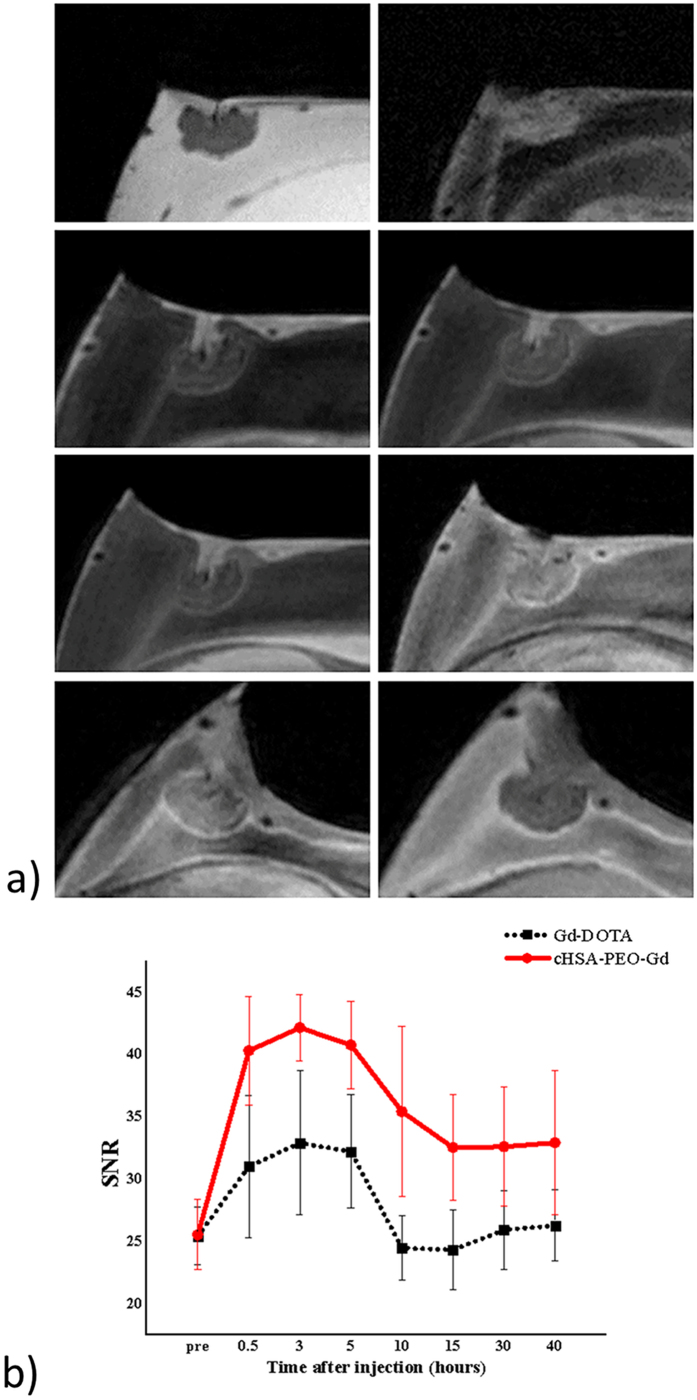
Representive MR images of cHSA-PEO(2000)_16_-Gd biodistribution in solid tumor tissuers (Left) and comparison of the SNR (Signal to Noise Ratio) of the tumor over the first 40 h after systemic injection of cHSA-PEO(2000)16-Gd and Gd-DOTA (Right).
